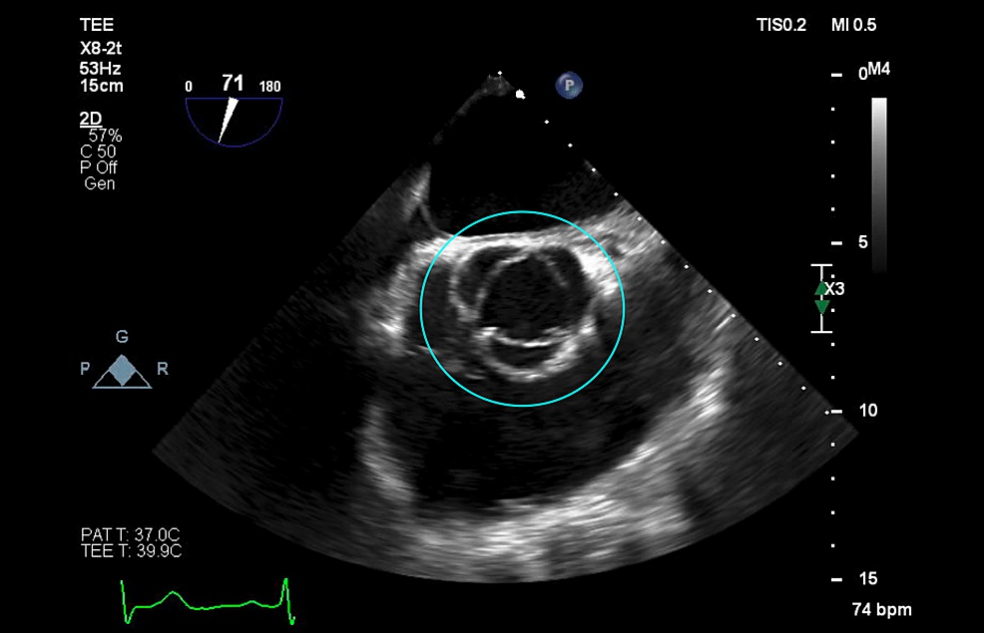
Mid-esophageal aortic valve short-axis view of transesophageal echocardiogram showing the aortic valve with no vegetation and open cusps (blue circle)
